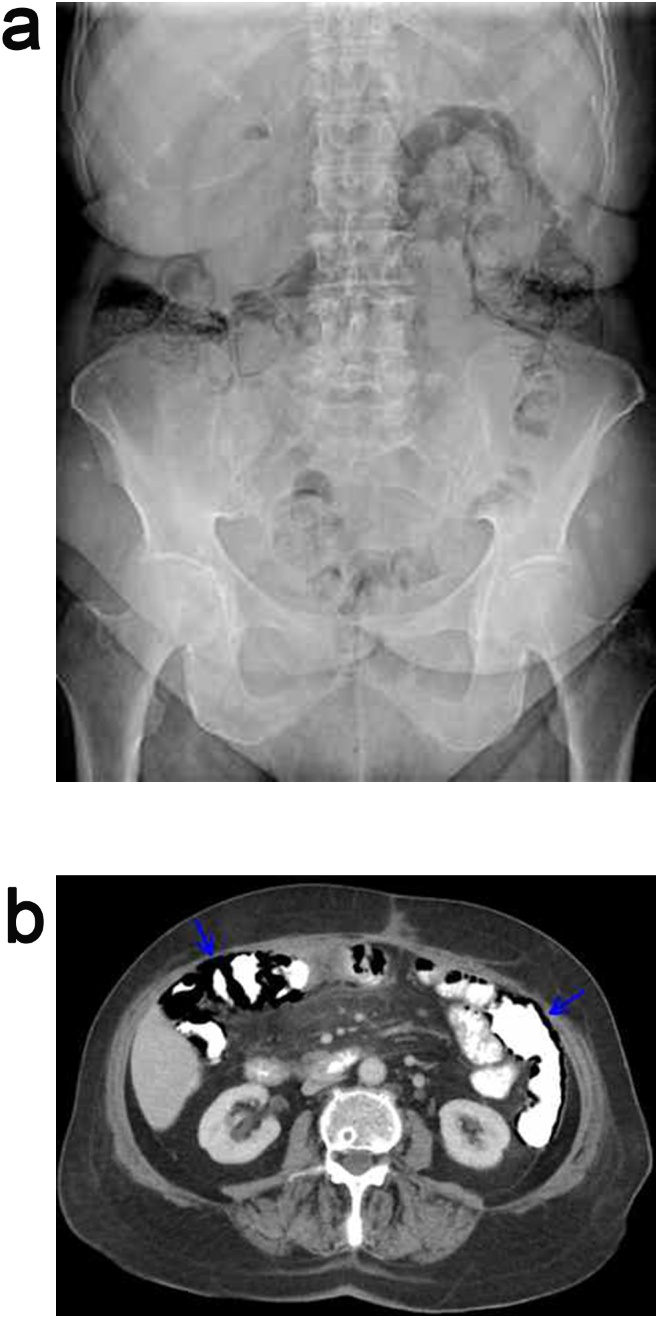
Abdominal (a) radiograph and (b) CT at initial presentation showing total linear submucosal and subserosal pneumatosis of the remaining colon with free intraperitoneal air.
